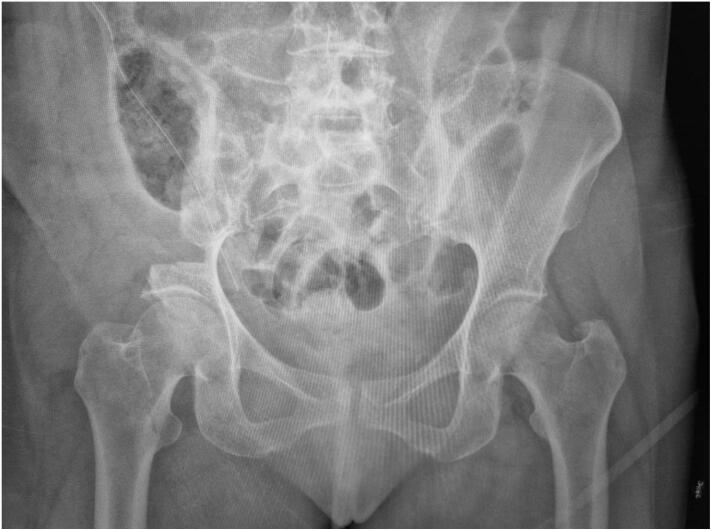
X-ray image of the patient post-tumor resection after 6 months.
